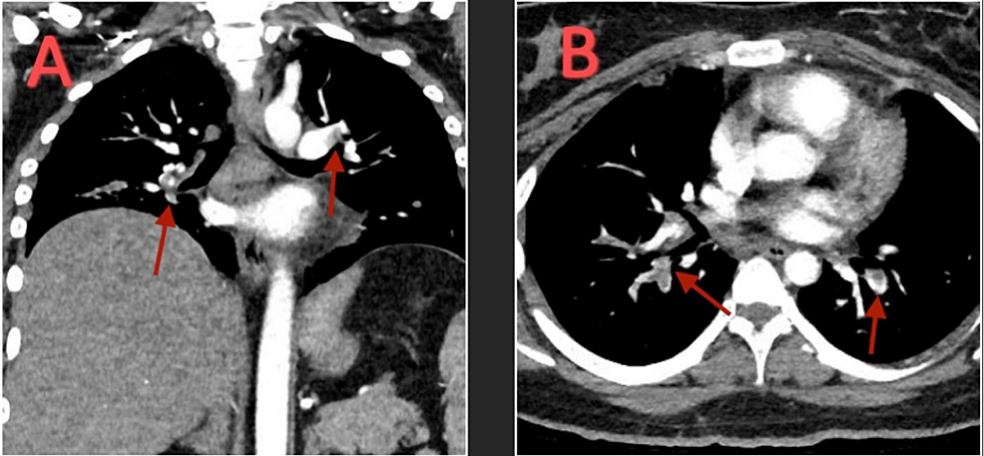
CTA chest with contrast. (A) Coronal view showing filling defects at the right interlobar artery and within the lobar and segmental branches of the right lower lobe. Left side filling defects with thrombus extending into the lobe and segmental branches of the lower lobe. (B) Transverse view showing similar filling defects. CTA: CT angiography.
